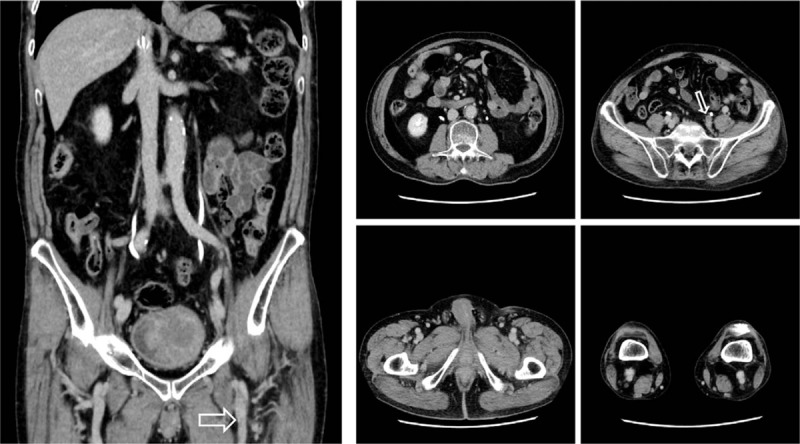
Abdominal computed tomography scan approximately 2 months after the initiation of treatment with both catheter-directed thrombolysis and factor Xa inhibitor. The arrows indicate reduced thrombosis.
